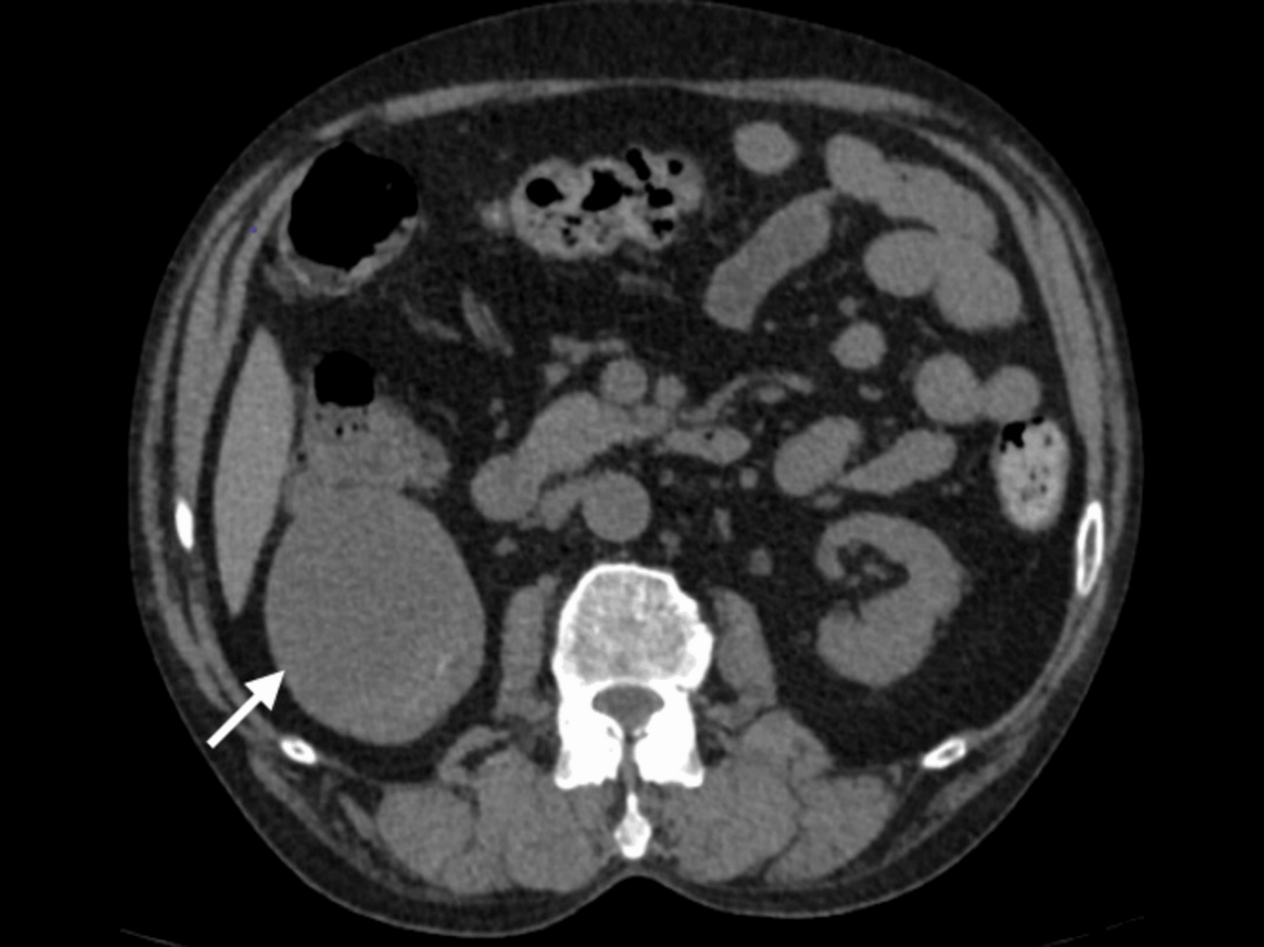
Axial contrast-enhanced CT demonstrates a well-defined cystic lesion with a peripheral location and homogeneous mural nodule enhancement (35 HU), and no perinephric fat stranding or lymphadenopathy.
